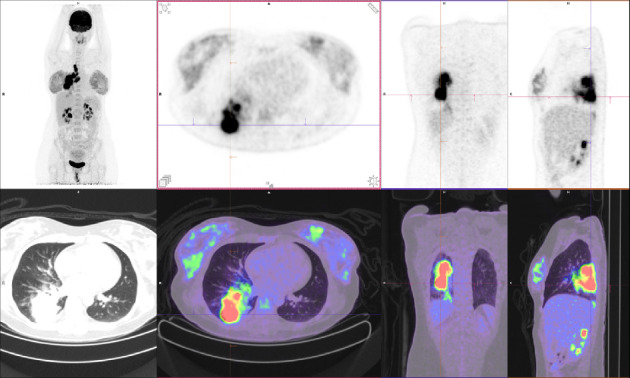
FDG PET-CT at diagnosis.
